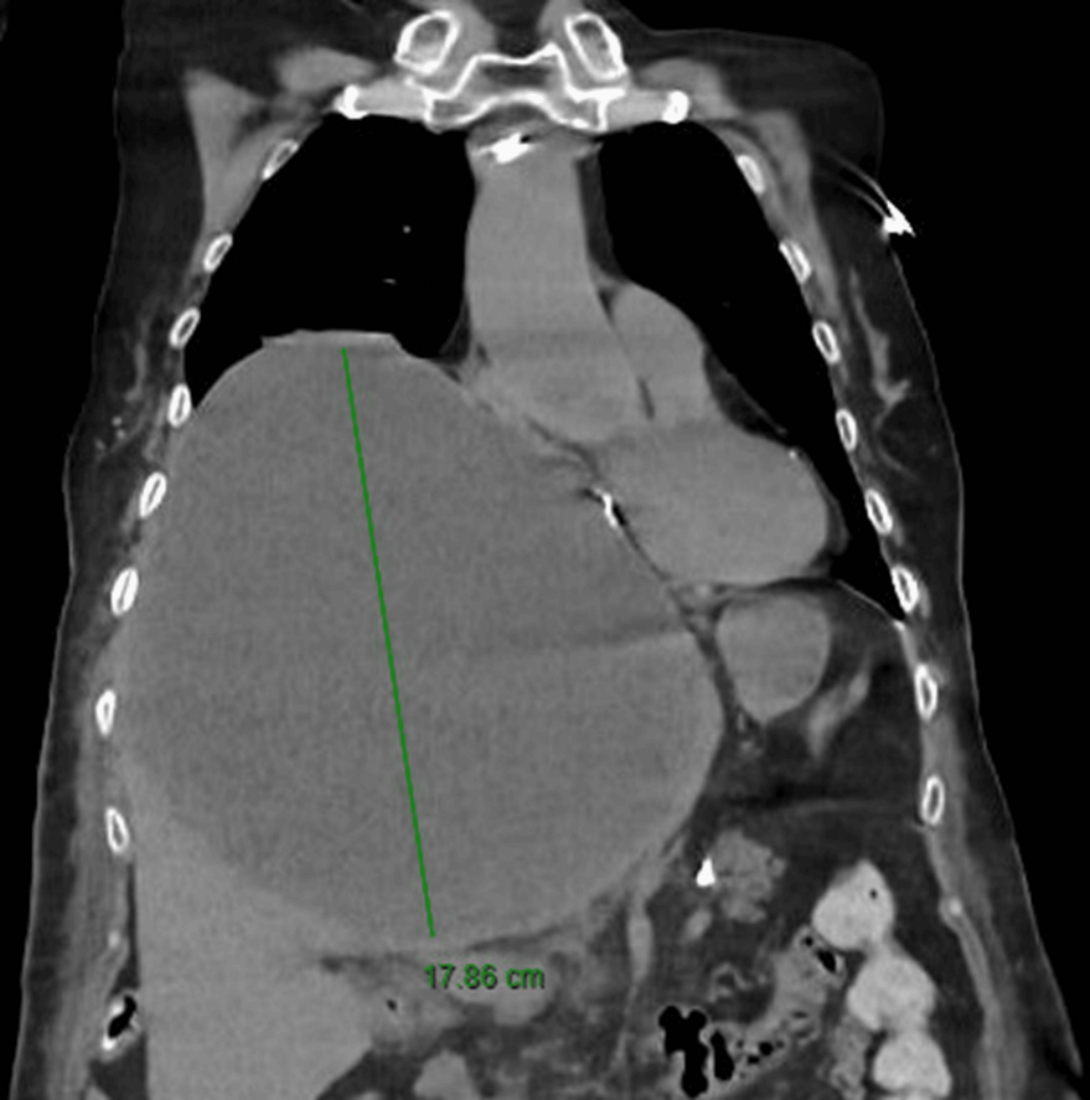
Coronal section of CT images displaying resultant mediastinal shift secondary to a large hepatic cyst measuring 17.86 cm
